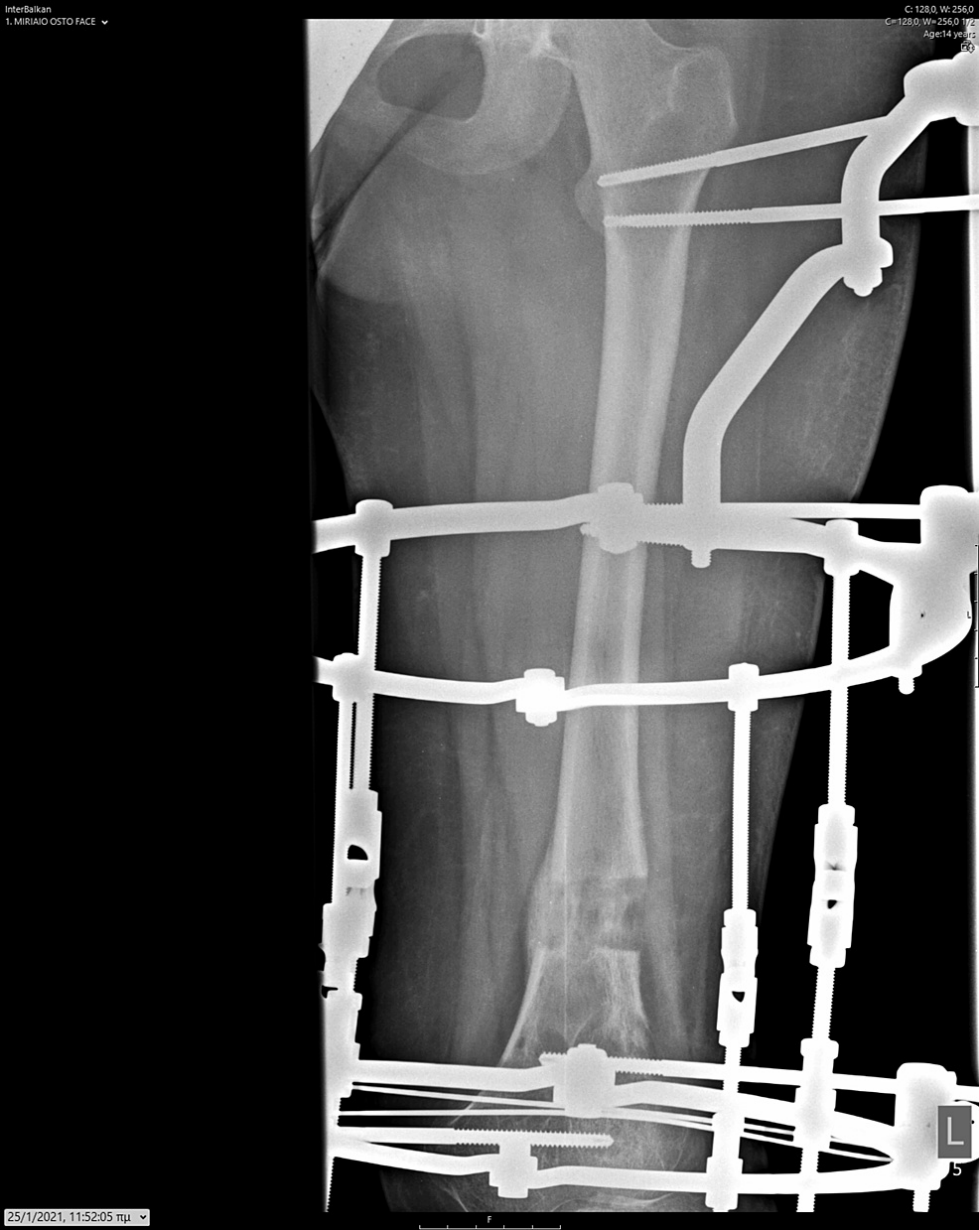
Antero-posterior X-ray at the conclusion of the corrective procedure for femoral valgus deformity, demonstrating adequate calcification of the newly formed bone.
